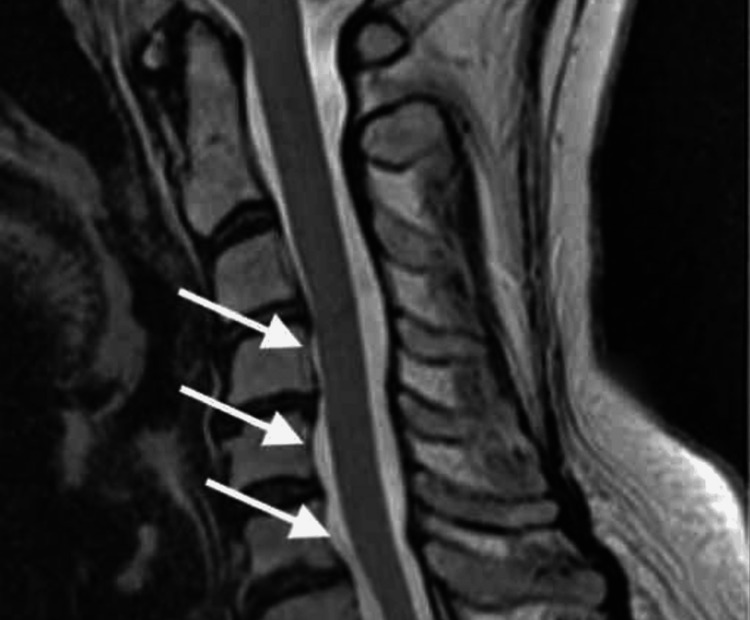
Cervical spine MRI MRI of the cervical spine demonstrating multilevel cervical spondylosis with moderate bilateral foraminal stenosis at C4-C6 (arrows). MRI: magnetic resonance imaging
